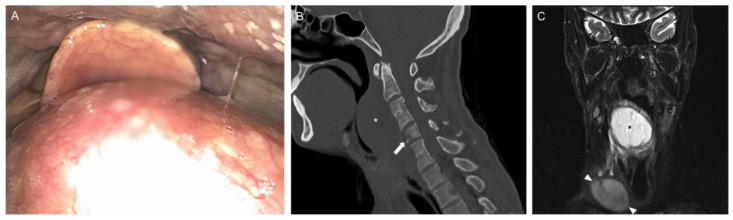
A 30-year-old male Somali refugee presented to the otorhinolaryngology outpatient clinic with an indolent 7 cm × 3 cm right supraclavicular mass. The patient reported a weight loss of 10 kg in 6 months, for which comprehensive diagnostic work-up for infectious/neoplastic etiologies had been initiated two months earlier. This had included an ultrasonographic evaluation and a cervical MRI, which confirmed the size and location of the mass suggestive of a lymph node conglomerate. An unremarkable chest X-ray and serologic screening for HIV, HSV, VZV, CMV, EBV, adenovirus, enterovirus, coxsackievirus, parvovirus, rubella, measles, mumps, hepatitis A, B, and C could not explain the lymphadenopathy. A core needle biopsy of the conglomerate showed granulomatous inflammation with a positive QuantiFERON-TB followed by negative mycobacterial culture/PCR. During a follow-up appointment where these results were discussed, the patient mentioned that he had developed two-week-long progressive hoarseness and subtle dyspnea. Flexible endoscopy revealed midline pharyngeal bulging pushing the epiglottis anteriorly (Panel A and Video S1). Cervical spine CT (Panel B, sagittal) showed the new retropharyngeal mass (5 cm × 3.5 cm, asterisk) and erosion of cervical vertebrae (mainly C5, arrow). As opposed to the imaging carried out two months earlier, the repeat cervical MRI (Panel C, coronal, STIR sequence) visualized both retropharyngeal abscess (asterisk) and supraclavicular conglomerate (arrowheads). Not all sequences could be obtained as the patient developed dyspnea during the MRI examination. Awake tracheostomy under local anesthesia, excision of the conglomerate, and repeated transoral/transcutaneous abscess drainage ensued. Positive culture/PCR confirmed pansensitive *Mycobacterium tuberculosis* and quadruple anti-TB treatment was initiated. The patient tolerated the therapy without adverse events and is doing well 6 months later with final orthopedic repair remaining. In retrospect, some of the vertebral lesions could have been observed in the initial cervical MRI carried out at an external diagnostic imaging center, but the local radiologist in private practice did not explicitly comment on them as the focus was the lymph node conglomerate. The primary infection for the hematogenous spread remains unknown. This case highlights that the differential diagnosis of TB has to be considered in all atypical head and neck pathology. Scrofula, i.e., tuberculous lymphadenitis in the cervical region, is one of the most common extrapulmonary manifestations of TB [1]. Response to antimycobacterial therapy is known to be slower than with pulmonary lesions, with novel treatment regimens yet to demonstrate efficacy [2]. In the case of abscess formation, different surgical options have been analyzed over many decades and have been found to be efficacious in combination with drug treatment [3]. Tuberculous spondylitis, or Pott disease, can lead to degeneration and even caseous necrosis of intervertebral discs, as can be seen between C3/4 and C6/7 above, or lytic destruction of vertebrae, as highlighted in C5 [4]. Globalization can result in the emergence and reemergence of infectious diseases such as TB in low-incidence countries [5]. Thus, knowledge of the signs and symptoms to establish a diagnosis is crucial to guarantee that patients receive adequate and timely treatment.
